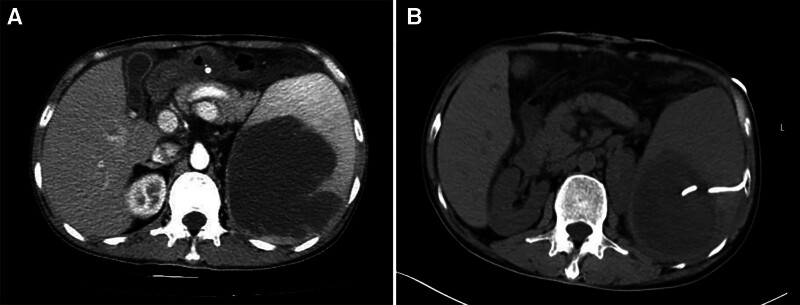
(A) Contrast-enhanced abdominal computed tomography image showing a low-density lesion in the spleen, and the lesion was considered to be a metastasis before splenic abscess formation. (B) Abdominal computed tomography image showing a drainage tube from the hole in the spleen.
